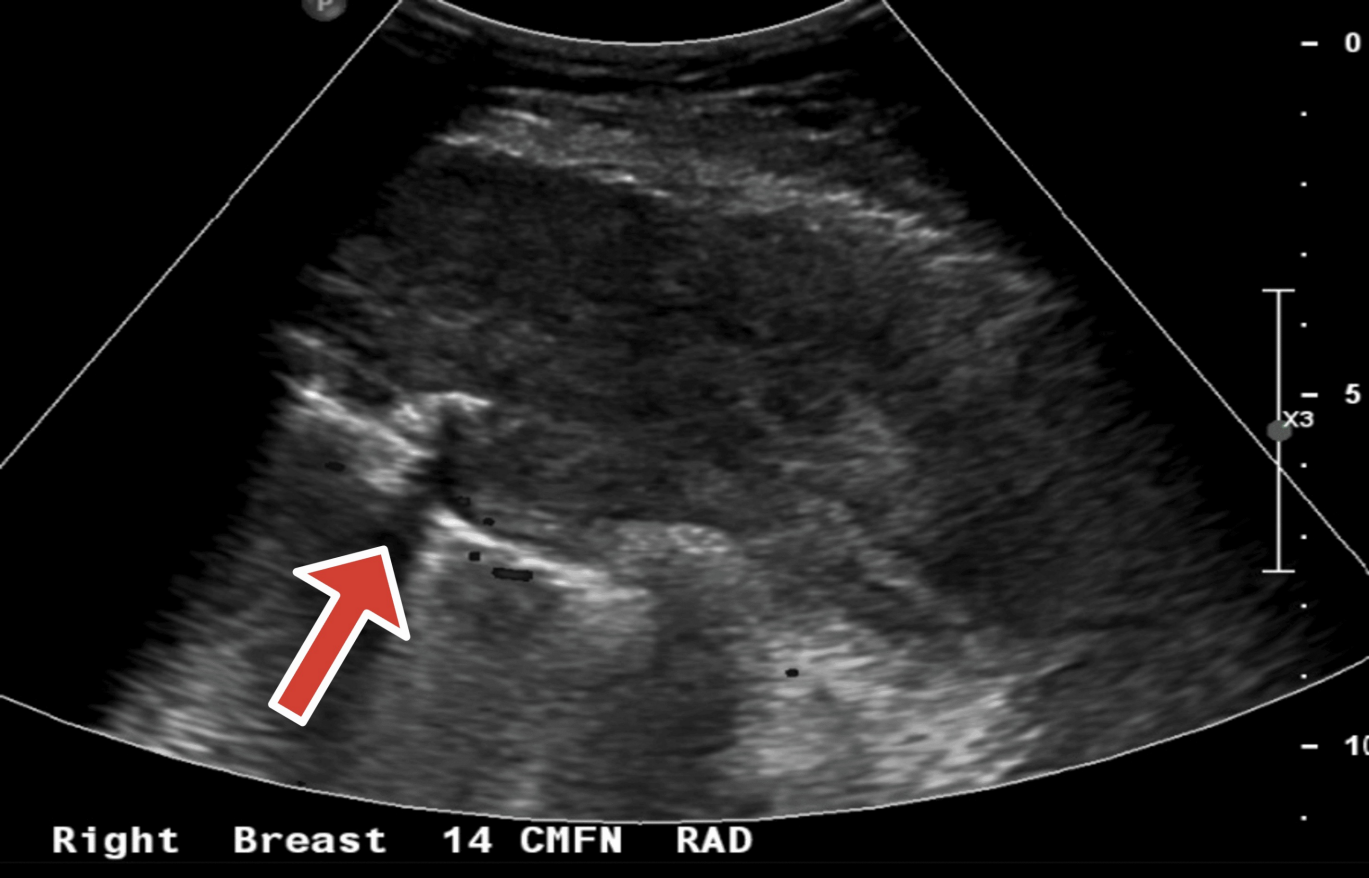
Ultrasound showing a heterogenous mass in the right anterior chest wall, extending to the subpleural space Arrow pointing at heterogeneous mass
